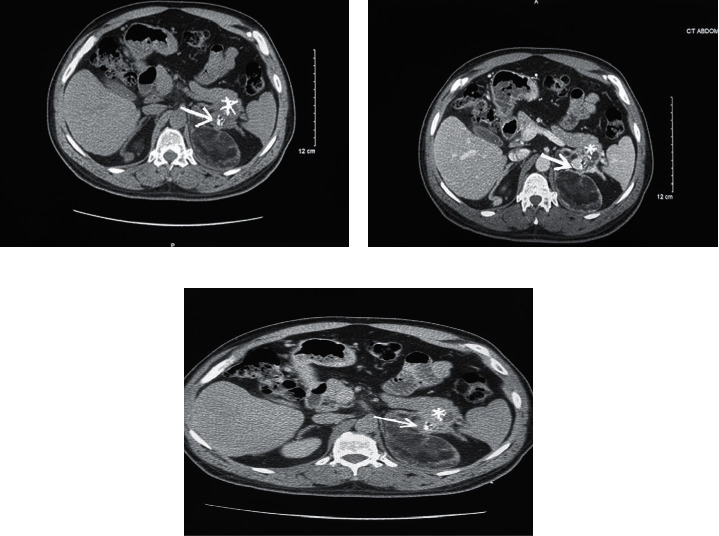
A multiphase CT with noncontrast (a), contrast (b), and delayed (c) images demonstrating the enhancing solid component of the left adrenal mass (arrow) and the region of questioned pancreatic invasion (asterisk).
